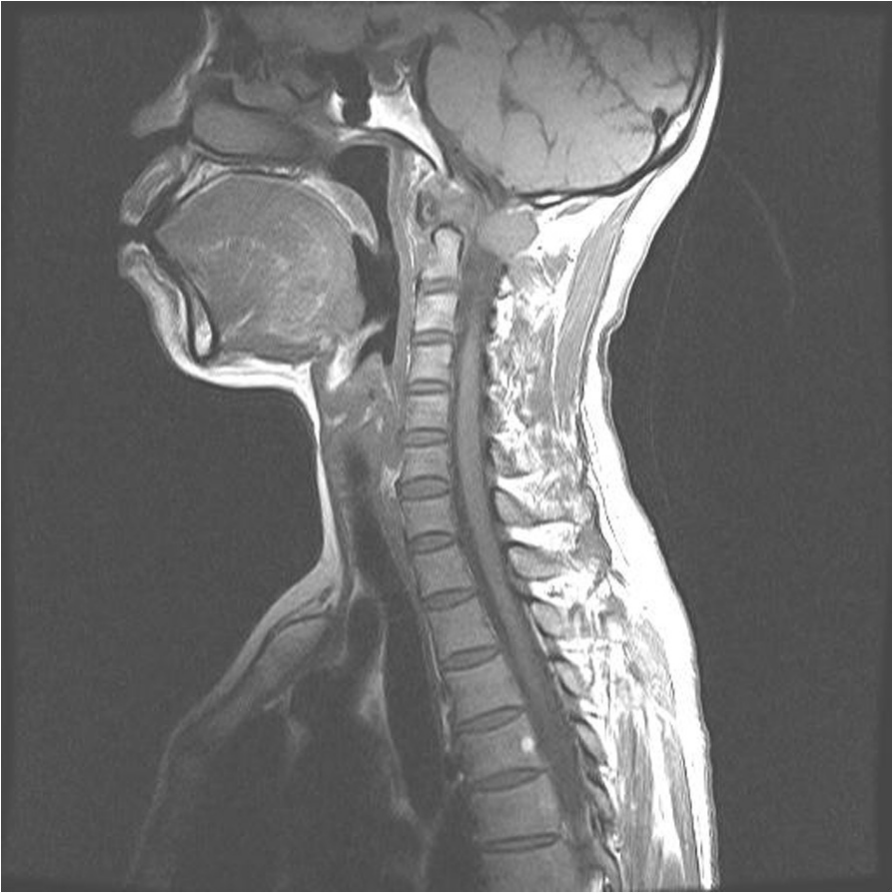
Sagital MRI preoperative image.
